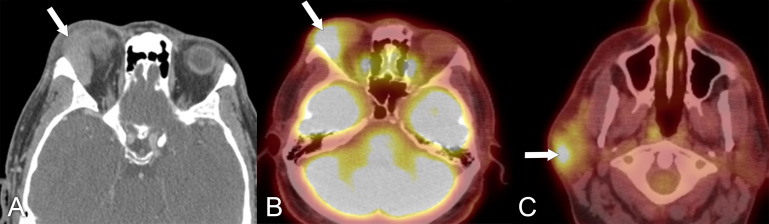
Imaging findings in a 49-year-old male patient who presented with right-sided proptosis and some orbital pain. (A) Axial CT with contrast, soft tissue window, shows right lacrimal gland enlargement (arrow). A biopsy of this lesion was performed, confirming the diagnosis of sclerosing orbital inflammatory syndrome, IgG4-related disease. (B, C) Axial PET/CT scans show increased uptake in the right lacrimal gland and in the right parotid gland, respectively (arrows).
